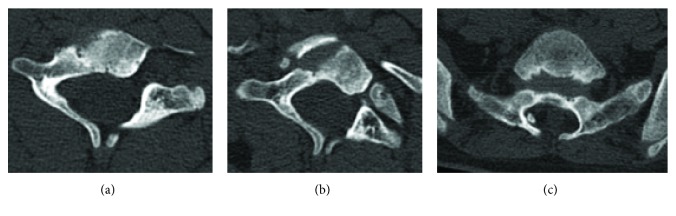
CT images showing spina bifida occulta in the (a) cervical, (b) thoracic, and (c) sacral spine of the patient (12 years).
